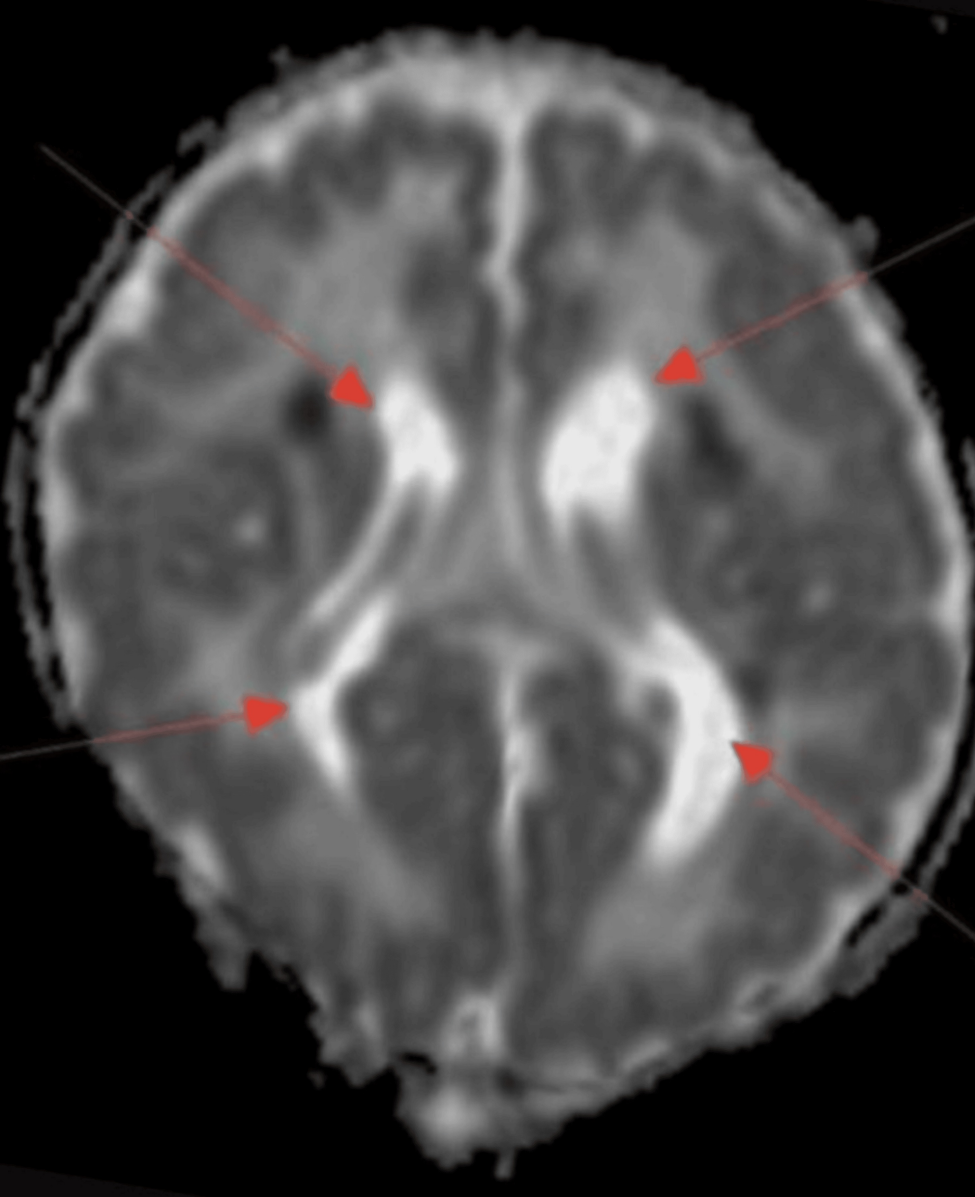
Brain MRI (axial view) showing excessive T2 hyperintense white matter (arrows) and volume loss in a periventricular distribution
